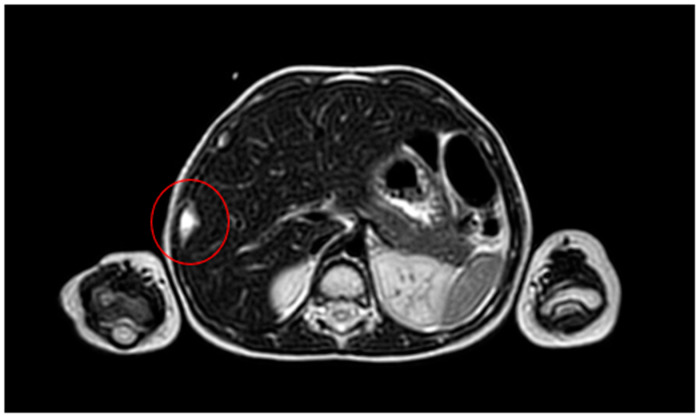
MRI at the end of the adjuvant CHT.
